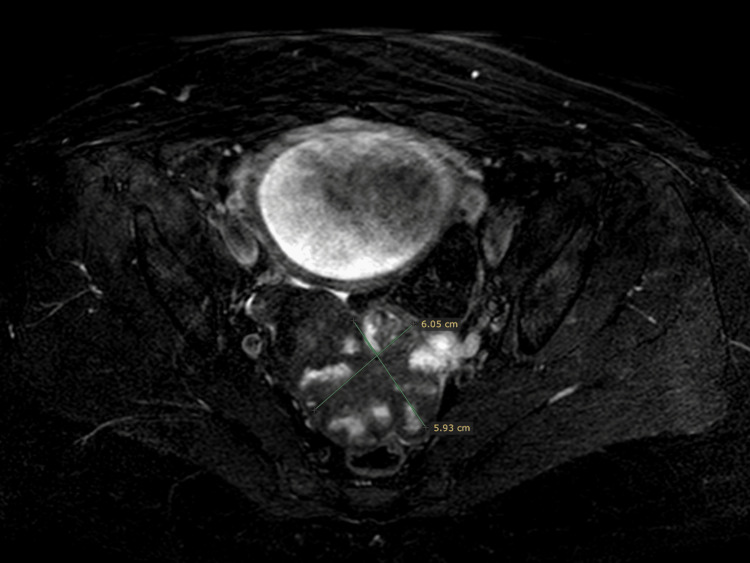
MRI at 28 weeks of gestation, dimensions of the tumor and location (axial plane) MRI, magnetic resonance imaging.
